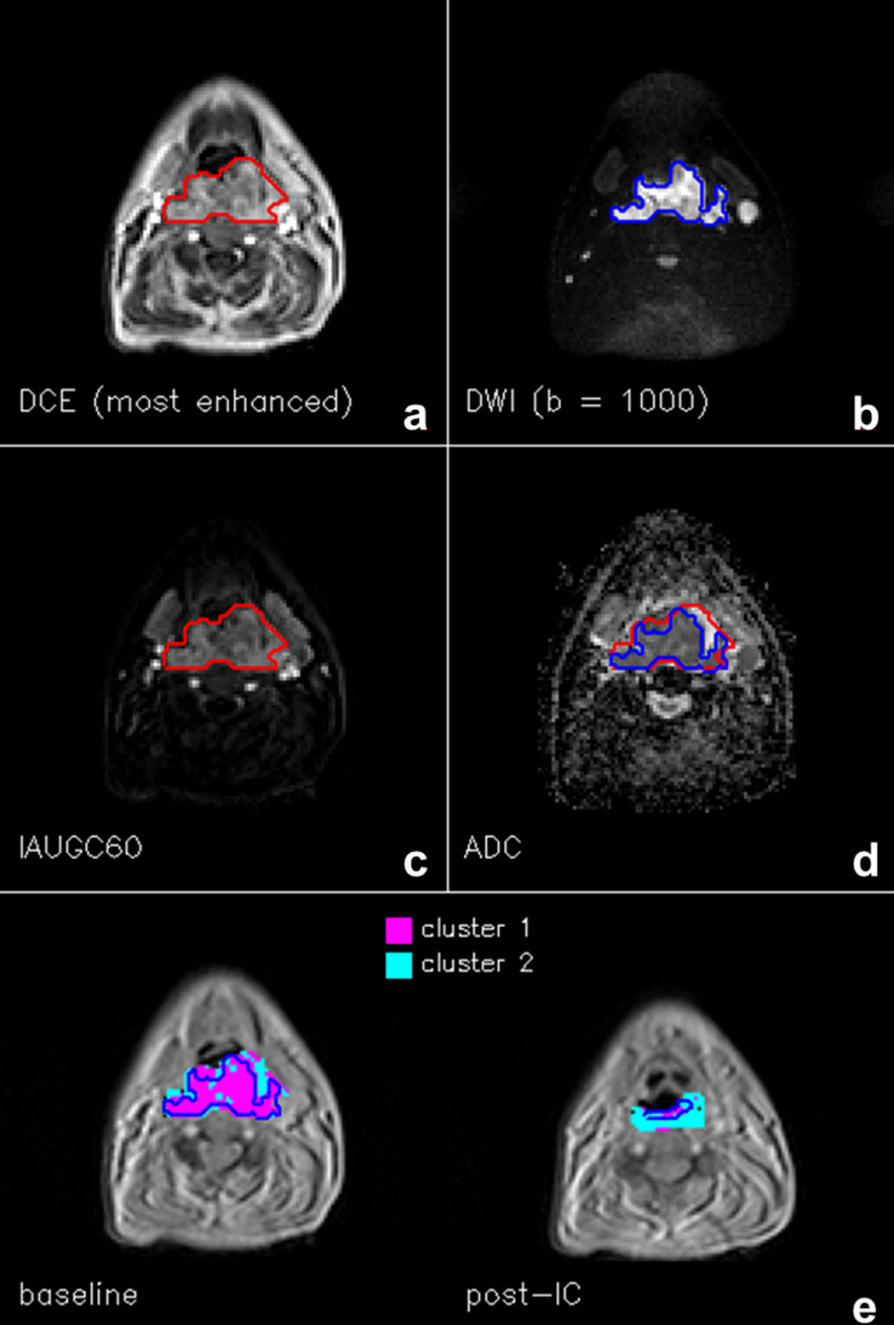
Slice with the largest tumour cross-section of a representative primary tumour. (a) ROI (red) contoured on the most enhanced frame of the DCE series, (b) ROI (blue) contoured on b = 1000 image of the DWI series, (c) IAUG60 map, (d) ADC map, with the two ROIs overlapped, and (e) cluster analysis map pre and post-induction chemotherapy (IC), in the simplest case of k = 2. The red ROI also encompasses the region where cluster analysis was performed; the blue ROIs represent the area of restricted diffusion independently contoured on DWI data, almost coincident with Cluster 1, which is characterized by both low ADC and high IAUGC60 values.
